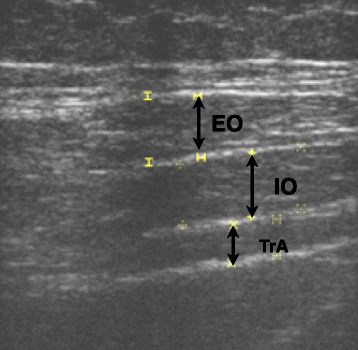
An ultrasound imaging of the lateral abdominal wall muscles taken during resting state. *TrA* transversus abdominis, *IO* internal oblique, *EO* external oblique
